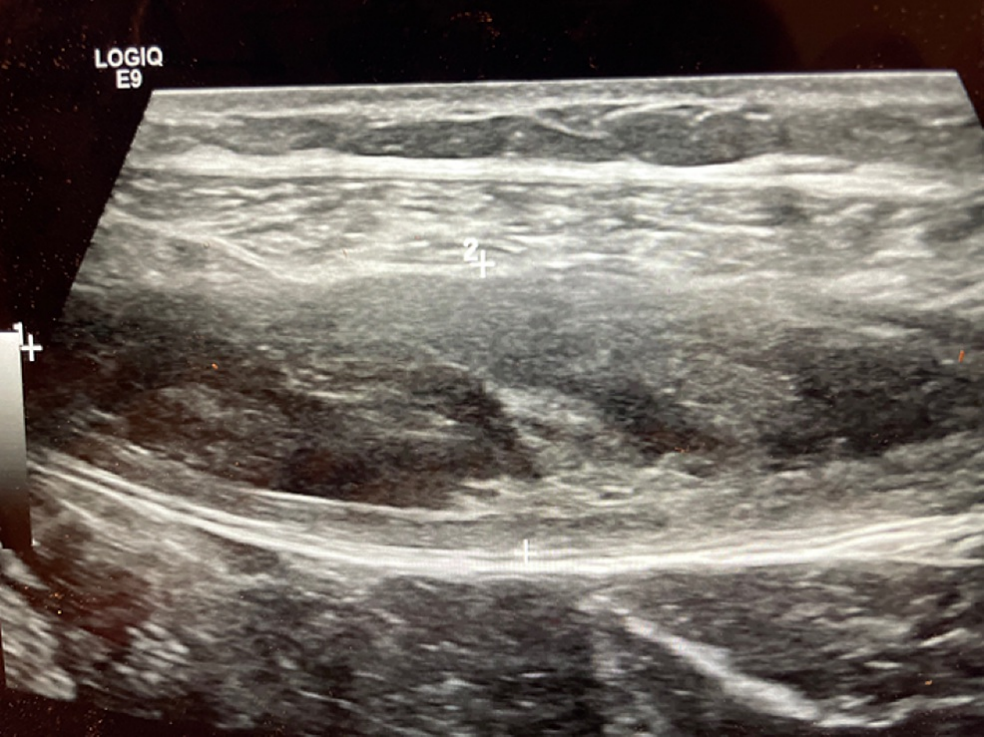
Ultrasonography findings demonstrating a rectus sheath hematoma between the anterior and posterior aspects of the rectus sheath fascia.
